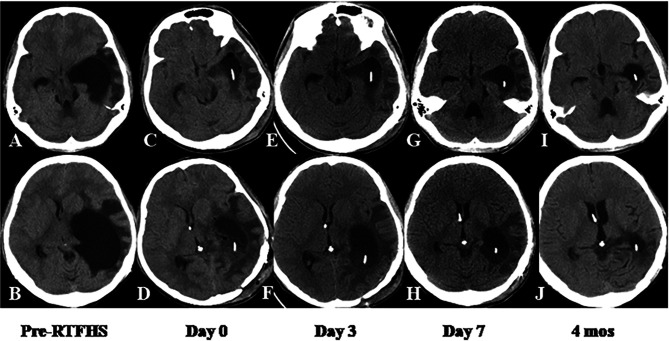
Case 6. CT scan showed immediate and further reduction of TTH volume at the level of midbrain (upper panel) and Foremen of Monro (lower panel). The volume and morphometric changes of TTH were shown before RTFHS (A, B), at Day 0 (C, D), Day 3 (E, F), Day 7 (G, H), and 4 months (I, J).
